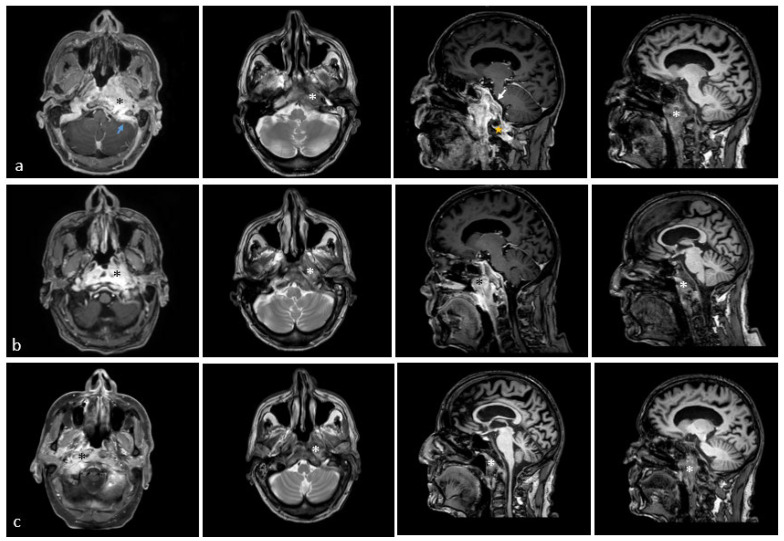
Exemplary representation of the MRI scans the patient received from April until June 2025. Axial images are represented on the left, sagittal images on the right. The first column of axial images consists of contrast-enhanced maximum intensity projection (MIP), the second column of axial images consists of native T2 images, the first column of sagittal images of b and c are contrast-enhanced compressed sensing T1 images, the sagittal image of c is a native compressed sensing T1 image, and the second column of sagittal images are native T1 images. (a) MRI scan from 9 April 2025 showing an extensive, malignant-suspicious mass lesion in the skull base/nasopharynx on the left side (black and white asterisk). Furthermore, broad-based involvement of the dura (white arrow) and ossary involvement/partial destruction and extensive perivertebral contrast accumulation caudally up to C2 (yellow star) are described, as well as partial sinus vein thrombosis affecting the left sigmoid sinus (blue arrow). (b) MRI scan from 30 April 2025 showing the known lesion with slightly regressive contrast enhancement but unchanged extent of the lesion (black and white asterisk). (c) MRI scan from 26 June 2025 showing the known lesion in the left nasopharynx, tending to decrease in intensity but with slightly increasing signal on the right side (black and white asterisk).
